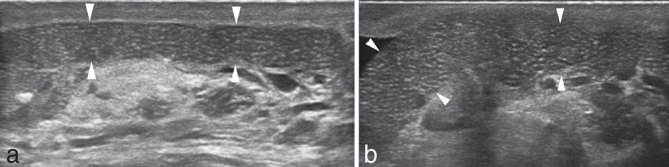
(a,b) On the external side of the left testis, an elongated, regular structure is seen (arrowheads), representing the migrated mesh. The regular, geometric echotexture can be noted. No acoustic shadow was seen. A very thin layer of fluid can be seen surrounding the mesh, although no clear signs of local inflammation can be seen. Power Doppler evaluation (not shown) was unremarkable.
